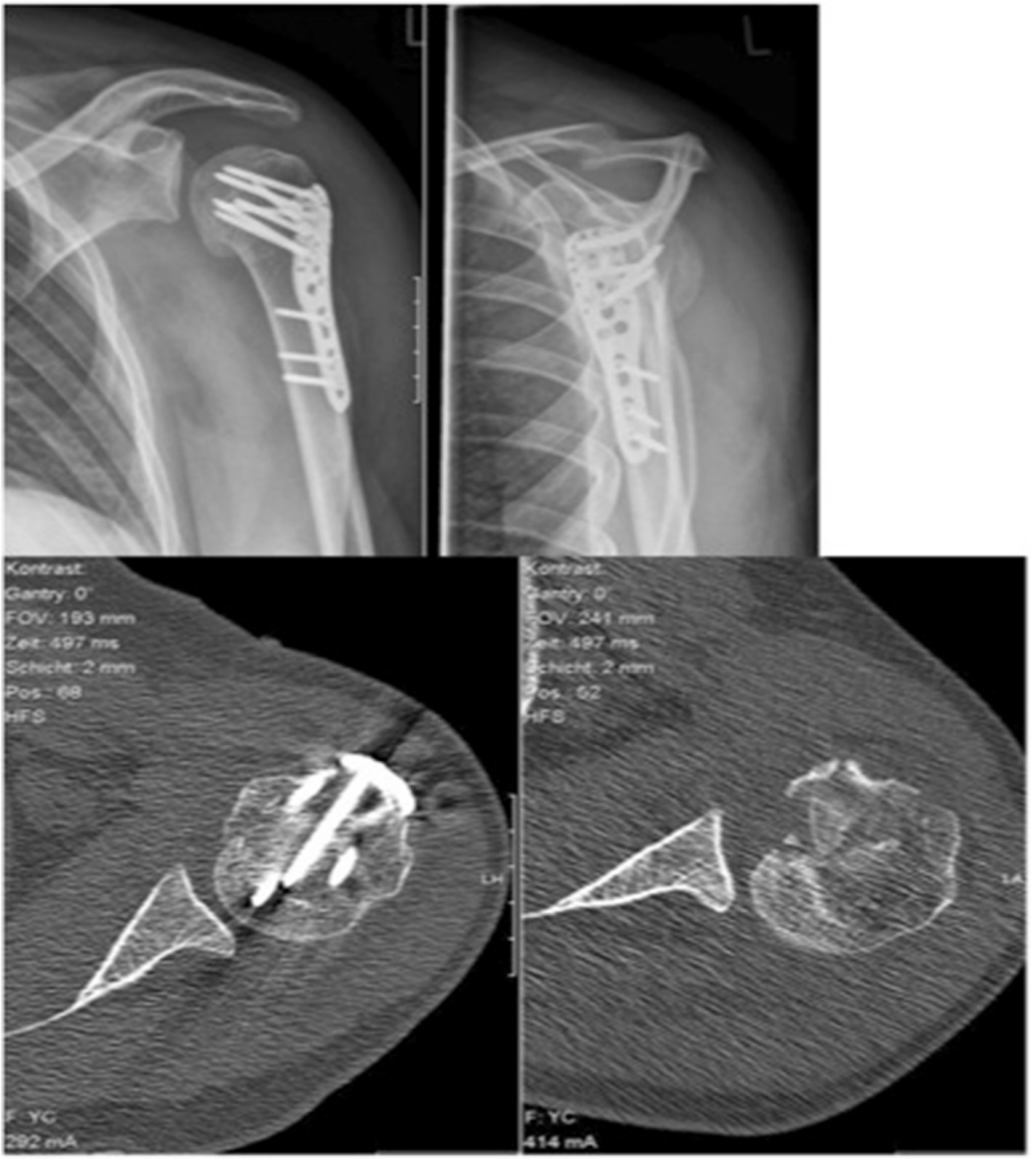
Post-operative x-ray and CT scan showing the restoration of spherical shape compared to pre-operative situation
